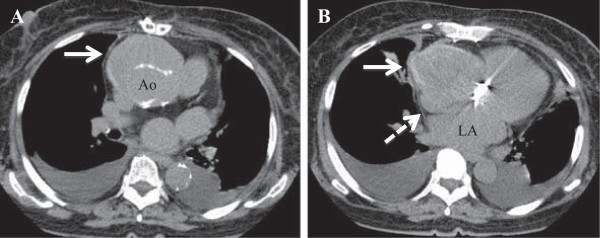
Computed tomography. (A) The pseudoaneurysm (solid arrow) anterior to the aortic root and right atrium. (A, B) Compression of the superior vena cava and right atrium (dashed arrow). Ao: ascending aortic artery, LA: left atrium.
